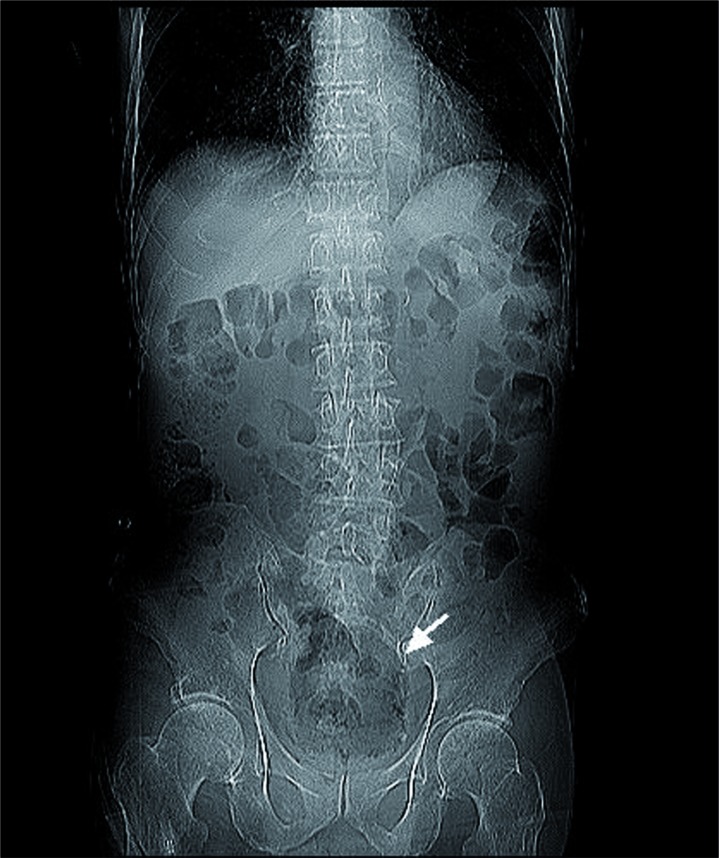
Radiography of the kidneys, ureters, and bladder revealed a low density over the whole bladder area and linear collection of gas along bladder wall (arrow).
